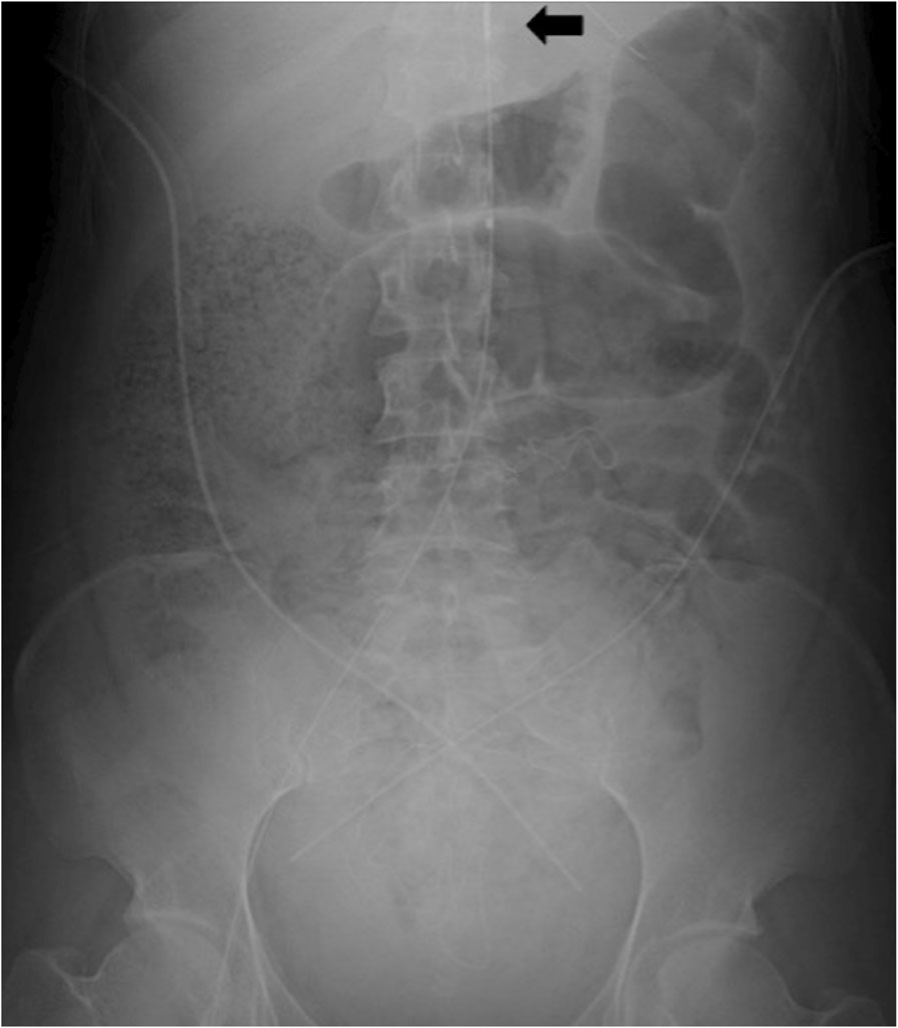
Balloon position was confirmed by abdominal X-ray in the operating room after surgery. The black arrow indicates the tip of the catheter placed at zone II of the aorta
